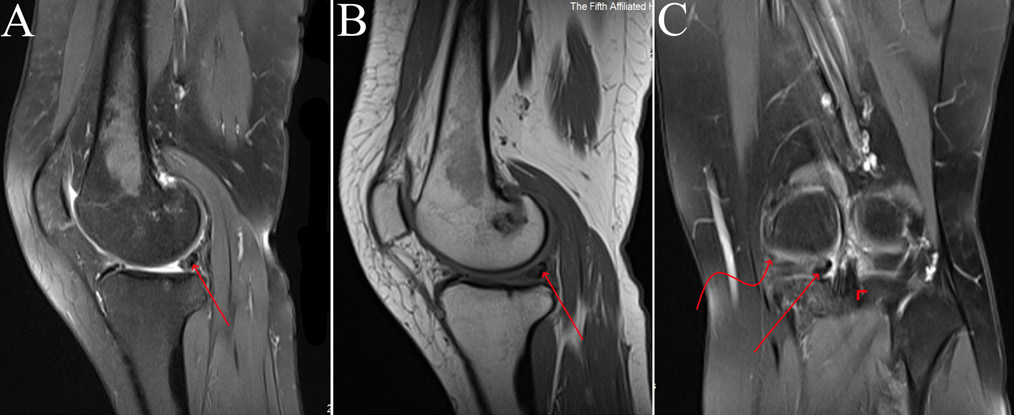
Sagittal MRI scan showing meniscal ossicle (straight arrow), medial meniscus (curved arrow) and posterior cruciate ligament (arrowhead). Note cancellous bone “inside” and cortical bone “outside” of the meniscal ossicle on (A).
